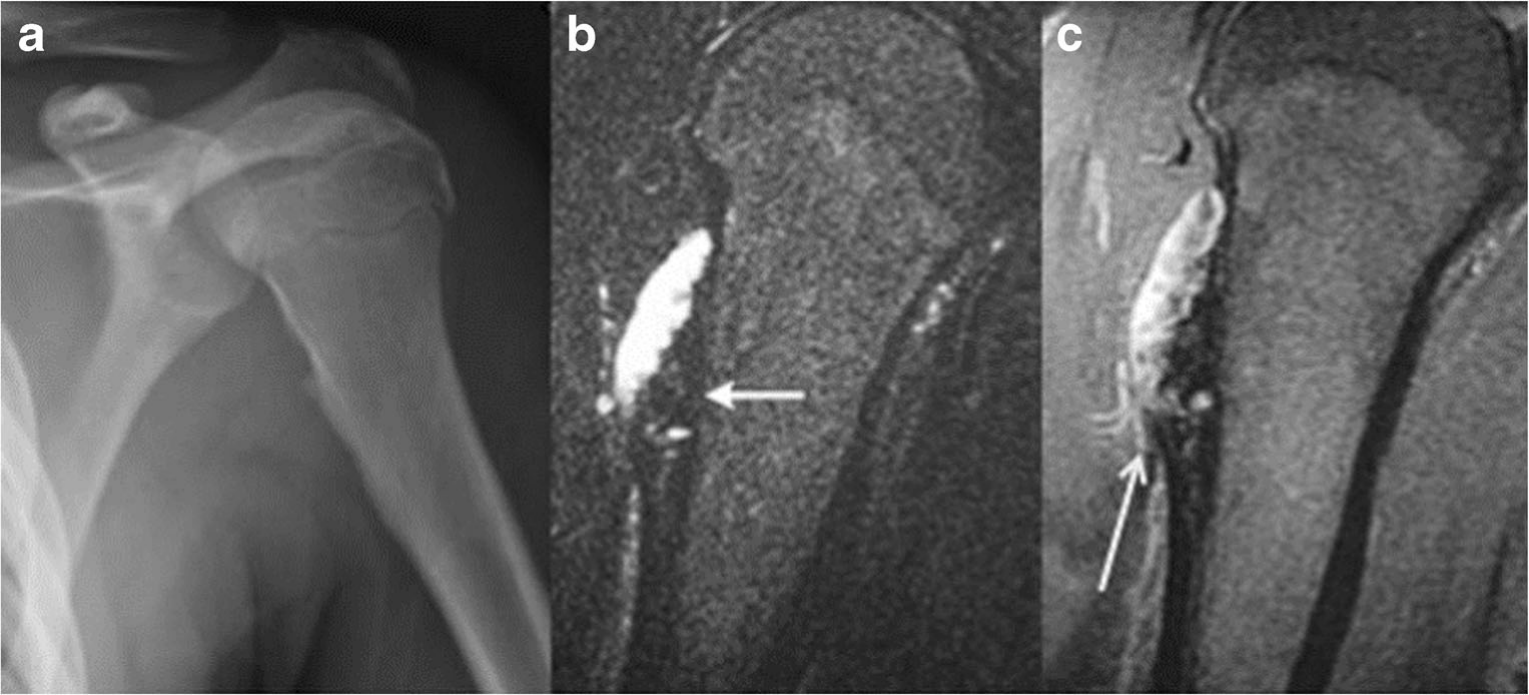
An 11-year-old boy with juxta-cortical chondroma at the proximal right humerus. A radiograph of the left shoulder reveals mild scalloping at the medial cortex of the proximal humeral metaphysis (*arrow*). A coronal T2 STIR image b and a coronal T1 post-contrast image c show a soft-tissue lesion abutting the cortex with a heterogeneously high T2 signal intensity suggesting cartilage (*thick arrow*), and predominantly peripheral contrast enhancement (*thin arrow*)Bizarre parosteal osteochondromatous proliferation (BPOP):BPOP, also known as Nora’s lesions, are rare, benign, reactive mesenchymal proliferations of bone adjacent to or attached to the outer periosteal surface of bone [13]. Proposed aetiologies include post-traumatic versus neoplastic. These commonly occur in the hands and feet (70 %), less commonly in long bones. Although mostly seen in adults, they are known to occur in children.These present radiologically as smooth and/or lobulated, variably calcified and/or ossified lesions, often with a broad base or stalk of attachment to an otherwise intact outer cortical surface of bone (Fig. 7). Lack of medullary continuity is the key differentiating feature from osteochondroma [14]. Remodelling of the adjacent bone cortex can occur. The lesion displaces but does not invade adjacent extraosseous soft tissues; adjacent soft-tissue oedema is seen with MR. High rates of recurrence have been documented post excision, in one study, up to 28 %[13].
